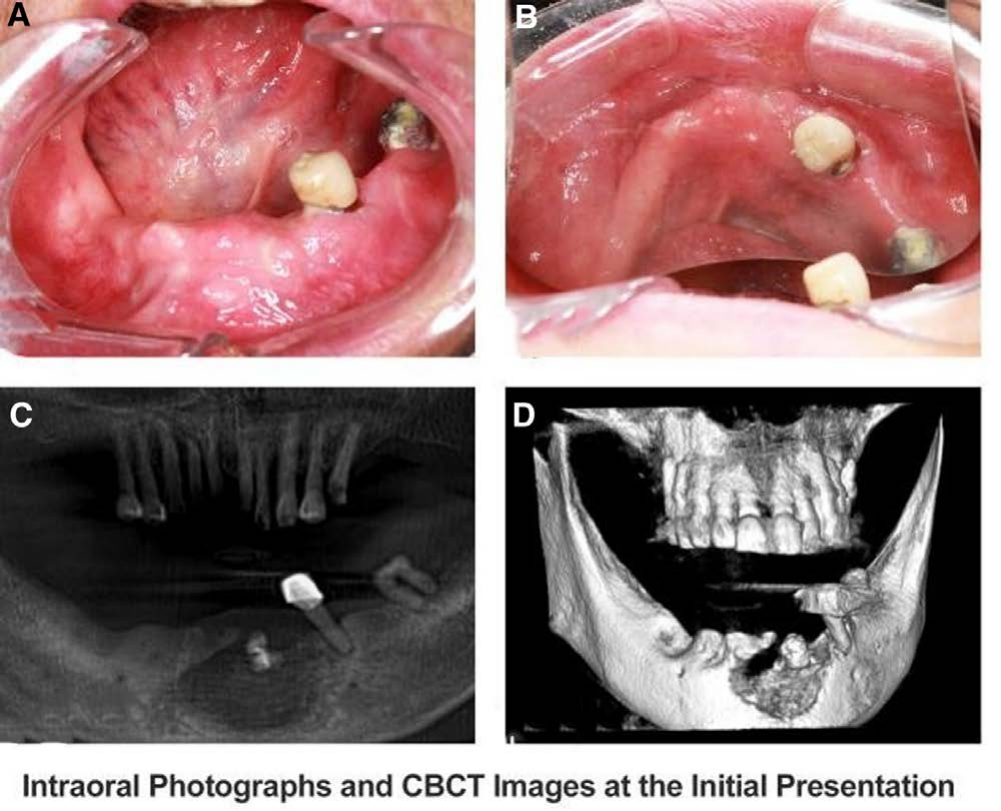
Photographs and CBCT images at the initial presentation. (A, B) Intraoral photographs showing the presence of a labial gingival fistula in the anterior mandibular region accompanied by abundant purulent discharge. (C) CBCT images revealed irregular, patchy low-density shadows in the anterior mandibular region, with peripheral areas displaying patchy areas indicative of necrotic bone. (D) Three-dimensional reconstruction showing cortical bone destruction with communication observed on both the labial and lingual aspects of the mandible. CBCT = cone-beam computed tomography.
